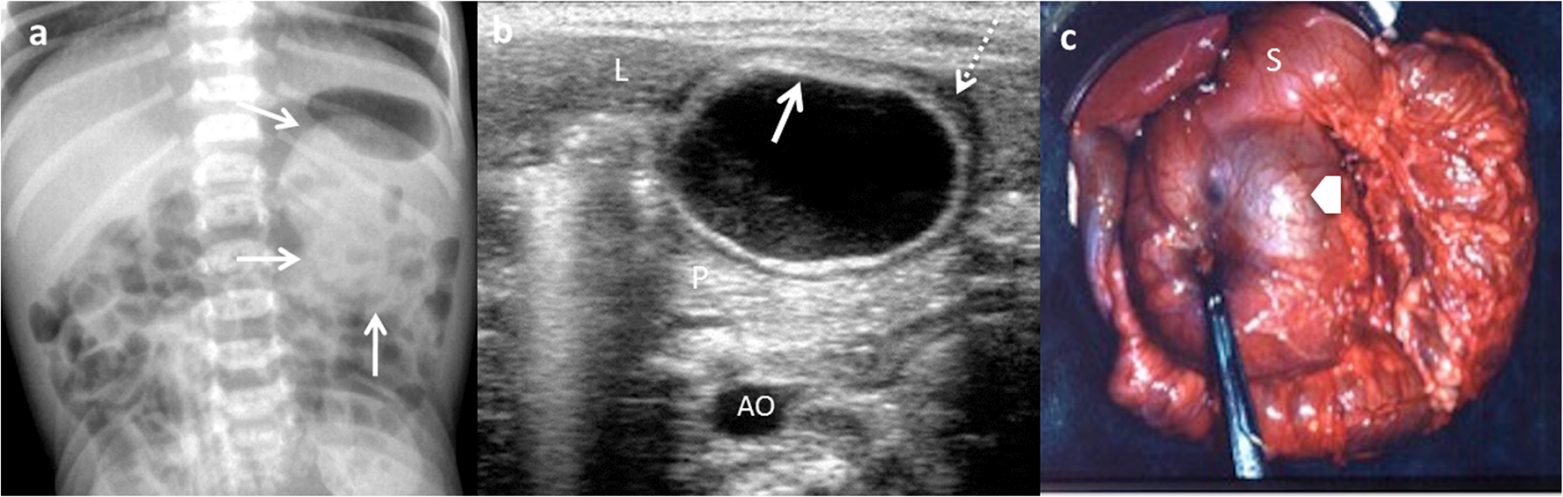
An 18-month-old girl with an abdominal mass in the physical exam is studied. a Abdominal X-ray: round, dense mass is discovered (*arrows*) in the left upper quadrant. b Transversal US view of the lesion: a cyst with the “double-wall” sign: the mucosa is hyperechoic (*arrow*) and the muscular layer is hypoechoic (*dashed-line arrow*). A gastric duplication cyst was suspected. *L* liver, *P* pancreas, *AO* aorta. c Laparotomy: antral duplication cyst was found (*arrow*). *S* stomach
